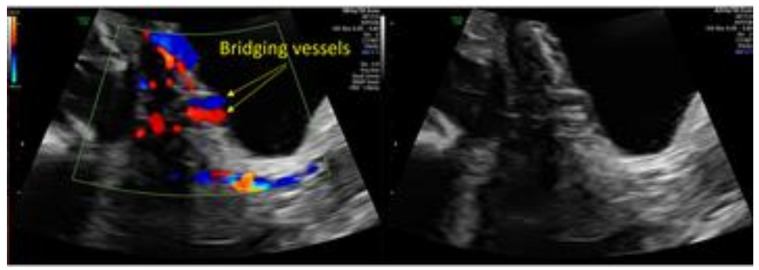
“Bridging vessels” interrupting the bladder wall in color Doppler ultrasound (left) and grey-scale ultrasound (right). Imaged adopted from Adu-Bredu et al. [36].
